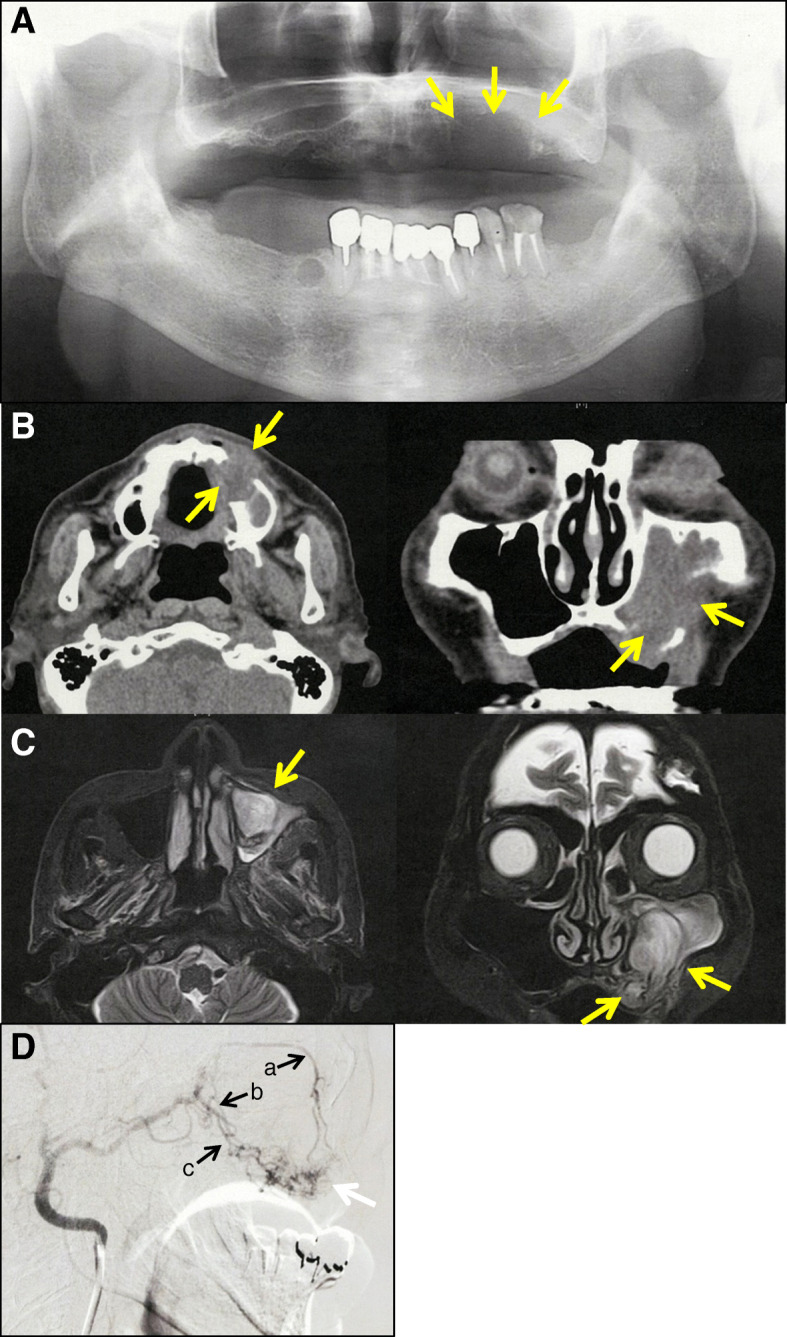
a Panoramic radiography revealed resorption of left maxillary alveolar bone (arrow). b Enhanced computed tomography showed a tumor destroying the left maxillary bone (arrow). c Magnetic resonance imaging showed a 47 × 31 × 22 mm mass in the left maxillary bone and maxillary sinus (arrow). d Computed tomography angiography showed a mass with strong enhancement (white arrow) fed by the infraorbital artery (a), sphenopalatine artery (b), and posterior superior alveolar artery (c)
